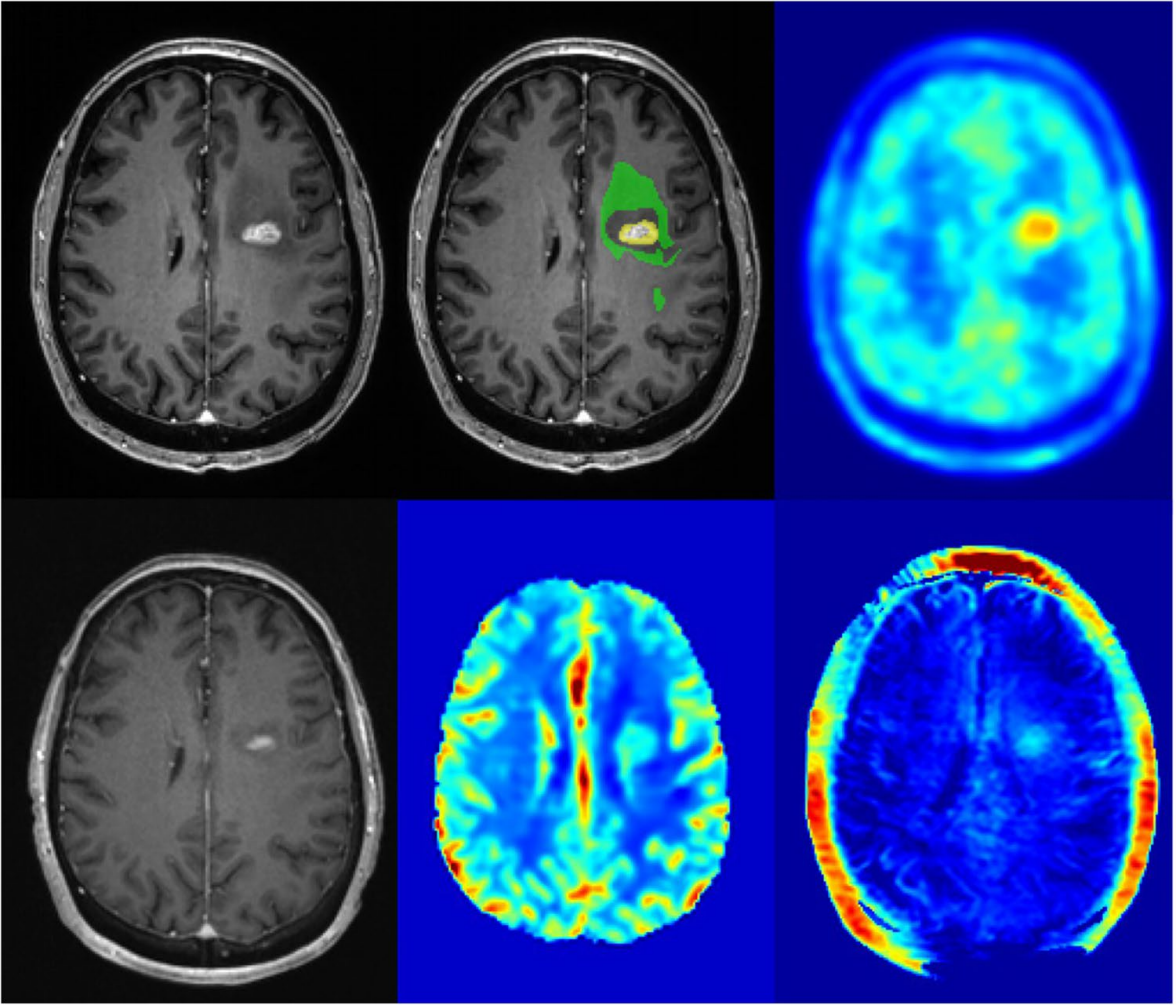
Example images of progressive disease in a 65-year-old female patient with left frontal GBM and a new contrast enhancement superior to the former resection area (upper left: ce T1-w, lower left: previous time point, upper middle: automated segmentation overlay (green: new FLAIR edema area; yellow: new ce area), lower middle: CBV, upper right: [^18^F]-FET-PET, lower right APTw)
